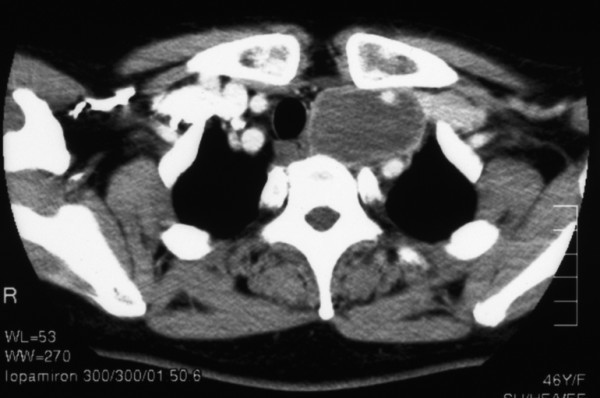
Chest CT showed a mass, measuring 5.0 cm in size in the upper mediastinum.
